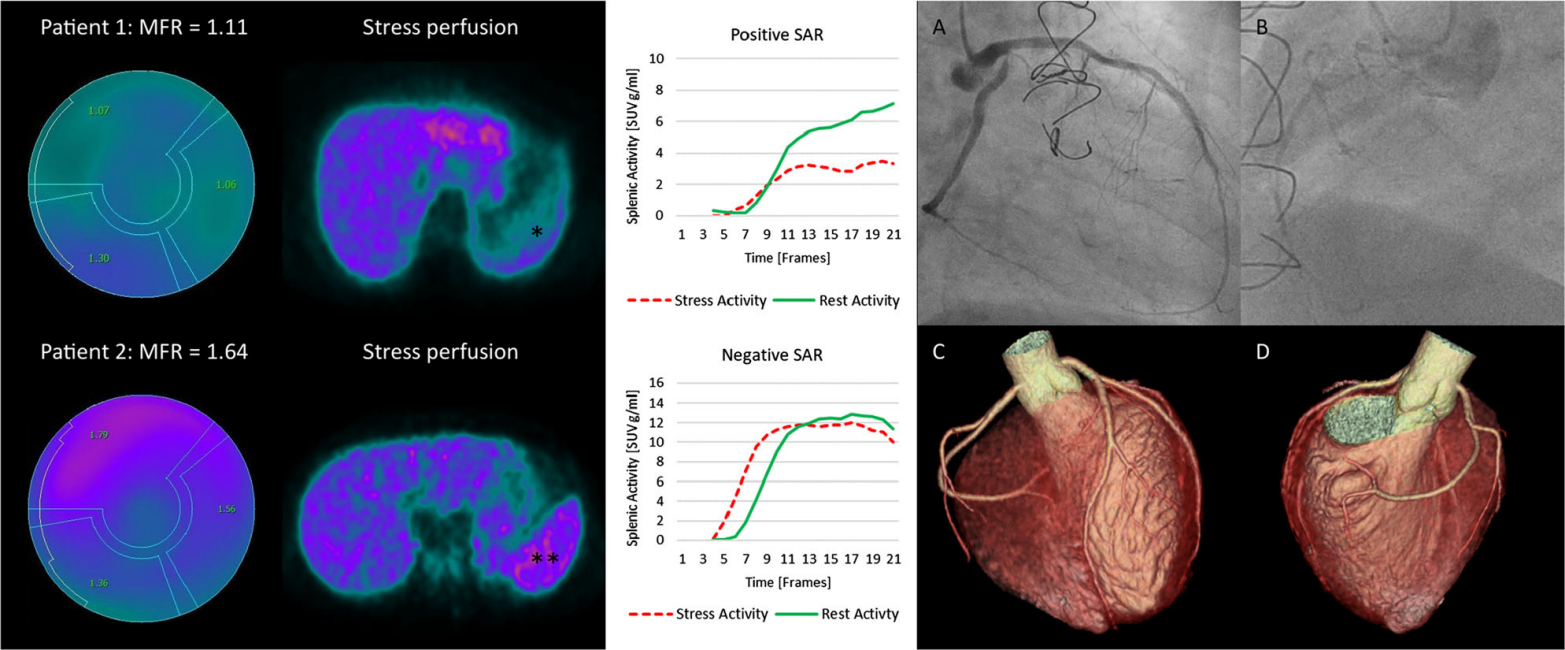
An example of two patients with impaired MFR. Invasive coronary angiography of patient #1 (top) with impaired MFR of 1.11 and a SAR of 0.41 (positive SSO: *) revealed severe CAD with a subtotal stenosis of the ostial left circumflex artery (A), occluded RCA (B) and subtotal stenosis of LIMA-LAD bypass graft, indicating truly reduced MFR. Coronary computed tomography (C,D) in patient #2 (bottom) with impaired MFR of 1.64 and a SAR of 0.93 (negative SSO: ) excluded obstructive CAD or calcifications, indicating failed adenosine response as the cause for the impaired MFR
